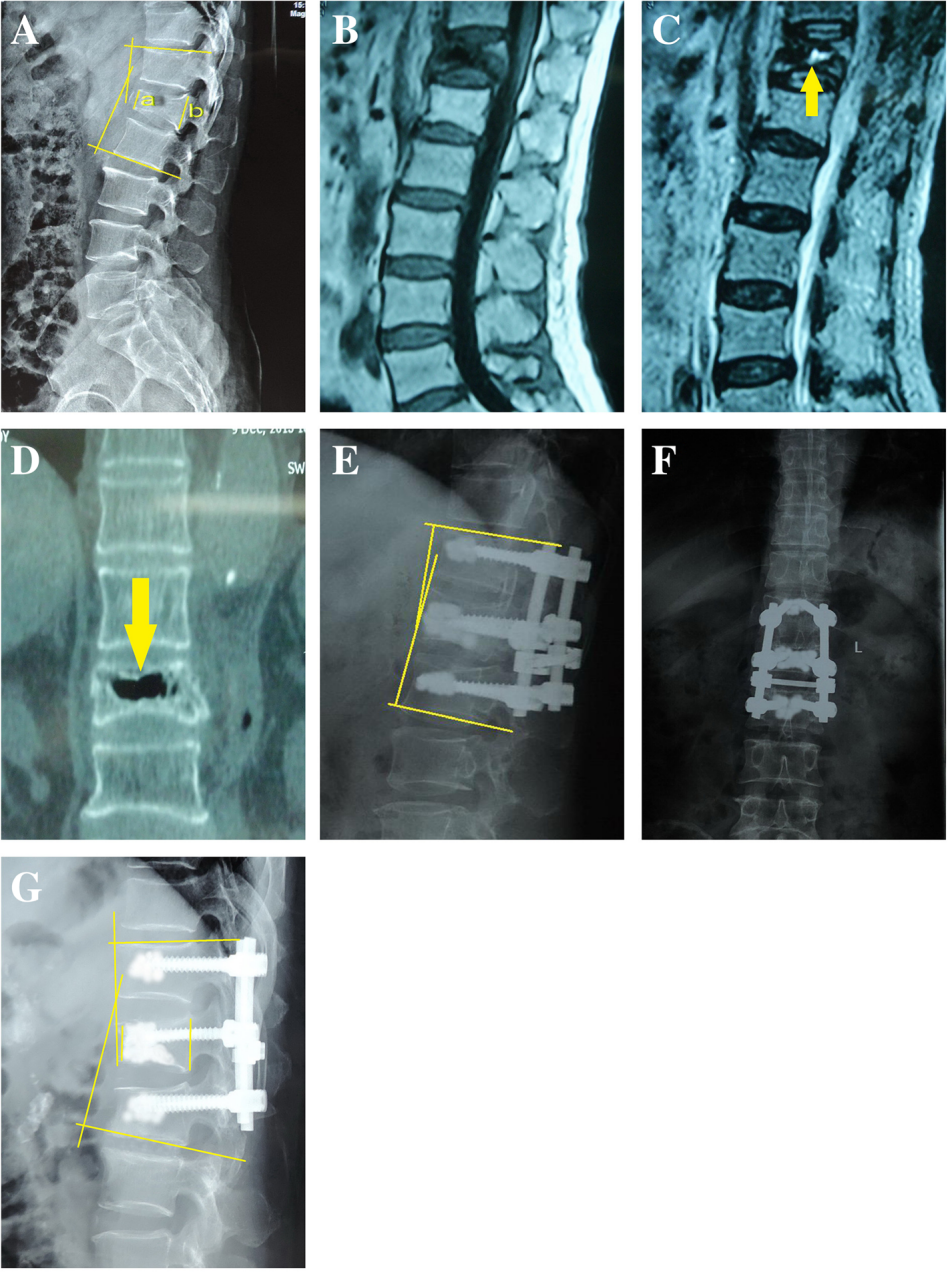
a: The measurement of kyphotic angle and average vertebral body height = (a + b) /2; b, c: local collection of fluid on MRI; d: sagittal CT reconstruction demonstrates the intervertebral cleft; e, f: at 3 days postoperatively, radiographs demonstrate correction of kyphosis and restoration of vertebral height; g: at 5 years postoperatively, radiographs demonstrate vertebral height loss in both the sabotaged and caudal vertebrae and an increase in kyphosis angle
